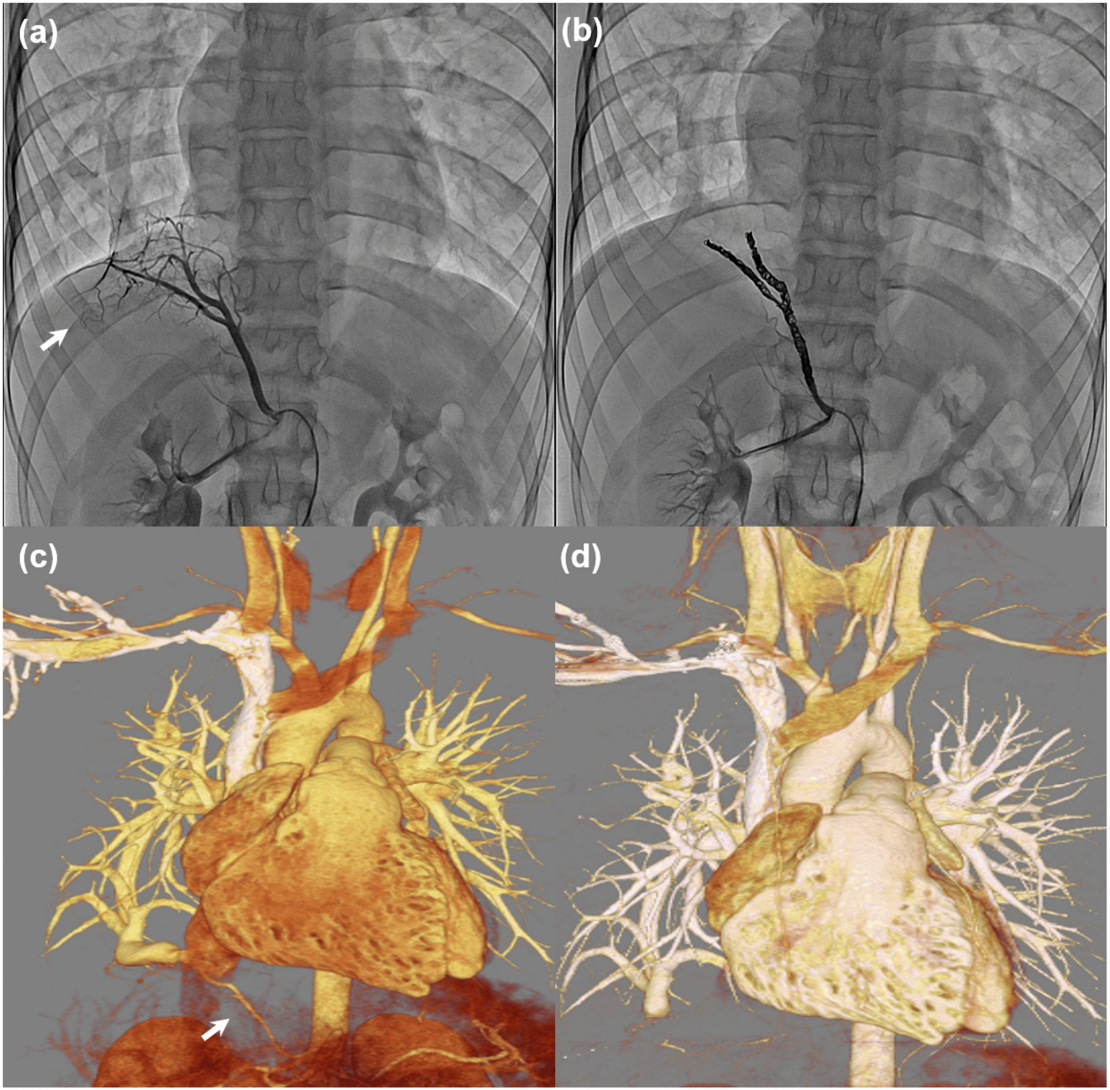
Angiography and 3D CT images of the patient at 19 years of age (a, b) Angiography images show an anomalous vessel originating from the right renal artery (a, arrow), which was blocked by coil embolization (b). (c, d) A 3D CT-based model of the patient's heart showed the scimitar vein (c, arrow), which is blocked off after the ligation (d).
